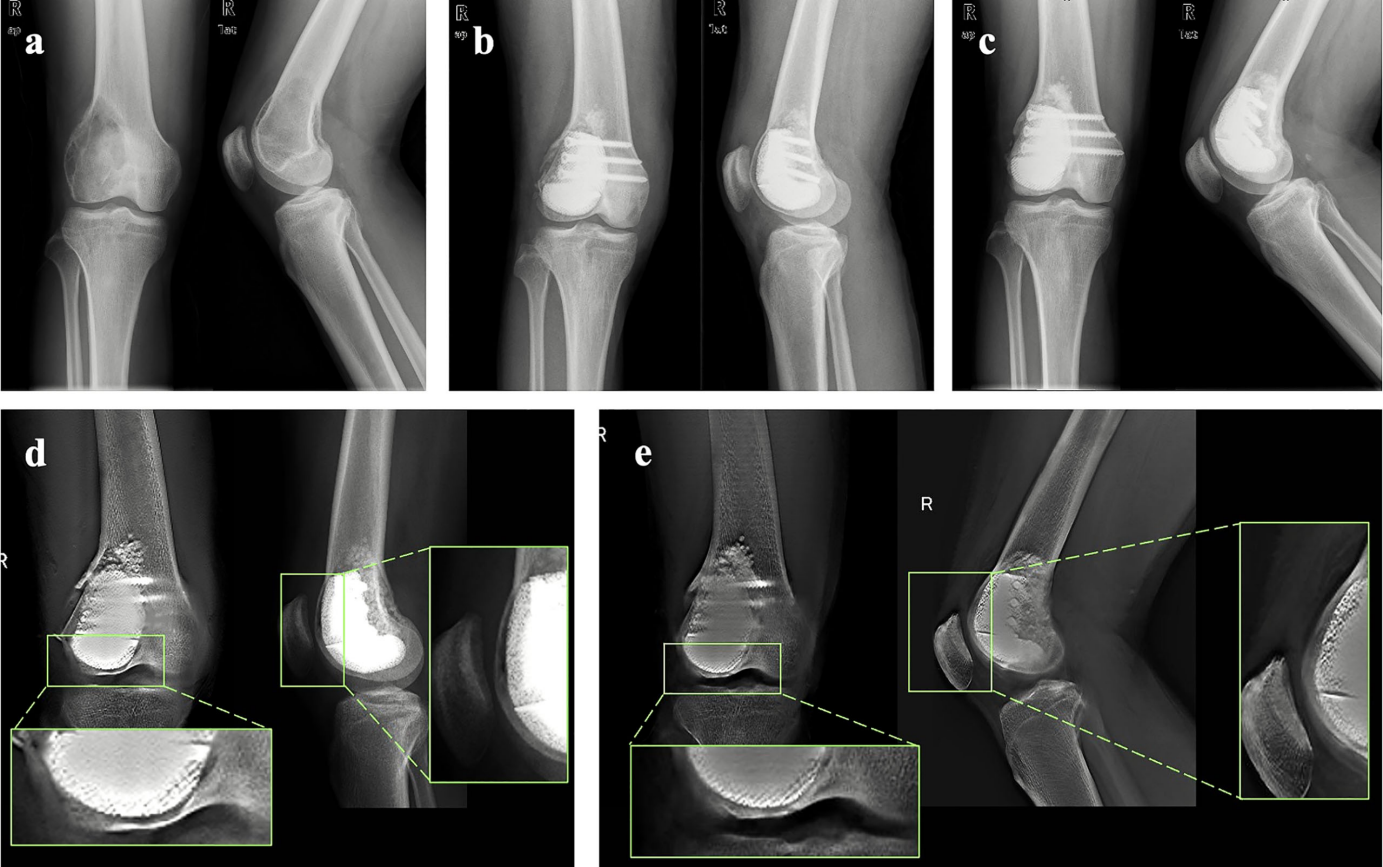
Representative case: (A) Preoperative X-ray of the knee of one patient from the cohort. (B) X-ray of the knee taken at 2 days after surgery. (C) X-ray of the knee taken at 24 months after surgery. (D) T-SMART taken at 1 day after surgery showed the interfacial gap between bone and implant (green box). (E) T-SMART taken at 24 months after surgery showed that the interfacial gap had disappeared (green box), indicating that excellent osseointegration was achieved.
